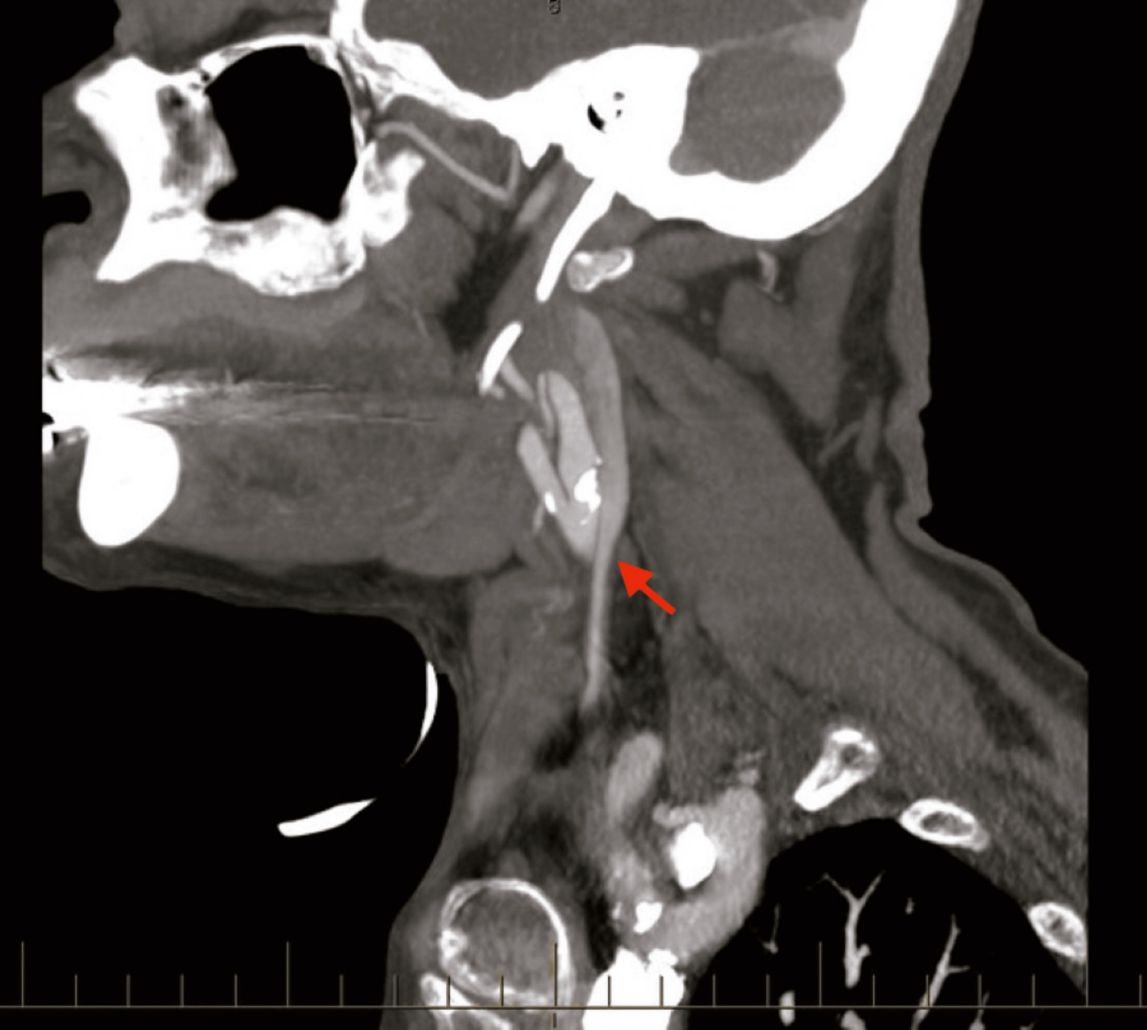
Sagittal section of CT angiography of the neck showing 70% stenosis of the left internal carotid artery as demonstrated by the red arrow Red arrow demonstrating 70% stenosis of the left internal carotid artery. CT: computed tomography
